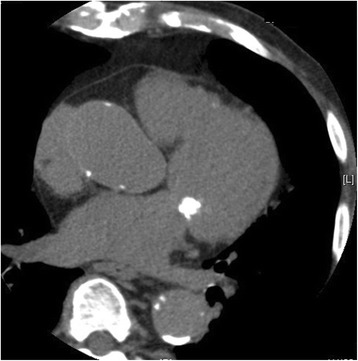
Cardiac computed tomography demonstrated a calcified cardiac mass in the mitral annulus with heavy mitral annular calcification
